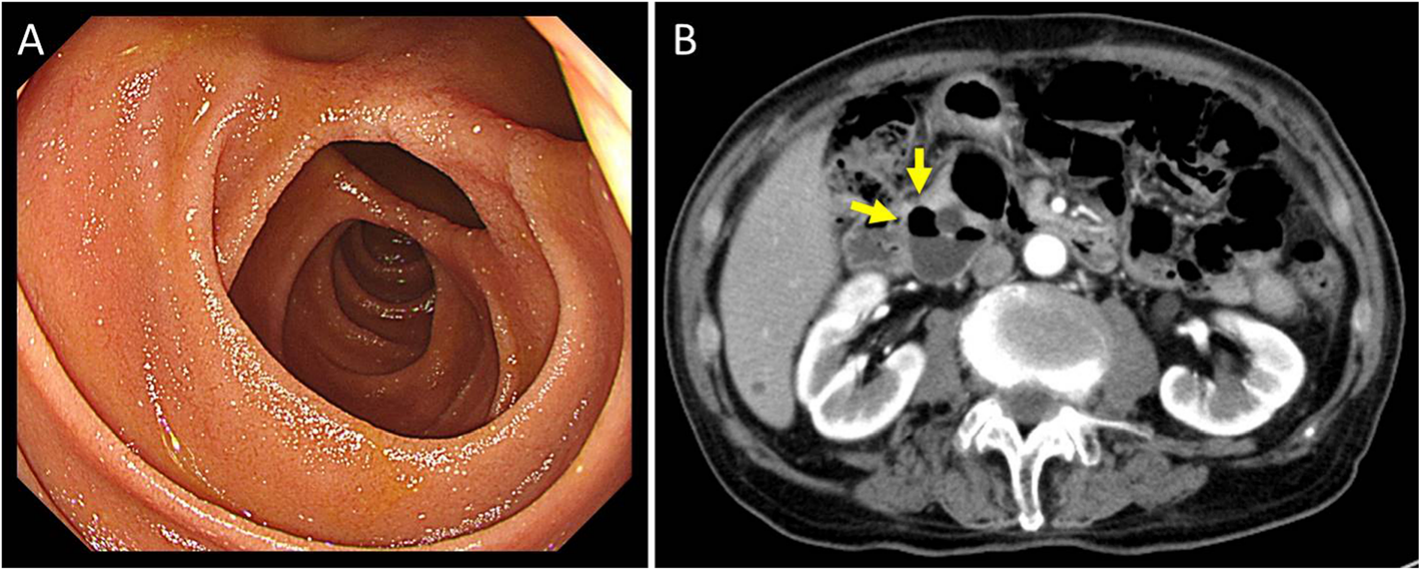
a The EGD showed two diverticula in the duodenal second portion. b CT showed a diverticulum in the duodenal second portion (arrow)
